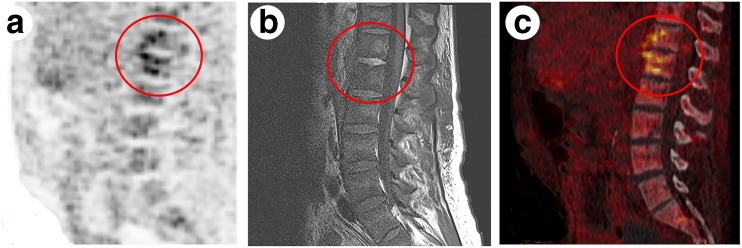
Imaging of the lumbar spine of a 43-year-old woman on haemodialysis who was admitted because of *S. aureus* bacteremia and back pain. ^18^F–FDG-PET (a) and ^18^F–FDG-PET/CT (c) showing increased ^18^F–FDG uptake of T12-L1 (score 4). T1-weighted Gd-chelate enhanced MRI (b) showing subtle enhancement of the intervertebral disc at level T12-L1 and of the perivertebral soft tissues and subtle interruption of the anterior endplates of T12 and L1 (score 4). The original MRI report was false-negative indicating a psoas abscess but no vertebral osteomyelitis. Original ^18^F–FDG-PET/CT and also reevaluated ^18^F–FDG-PET/CT and MRI did show vertebral osteomyelitis on level T12-L1. Repeated MRI after 2 weeks also confirmed the diagnosis of vertebral osteomyelitis
